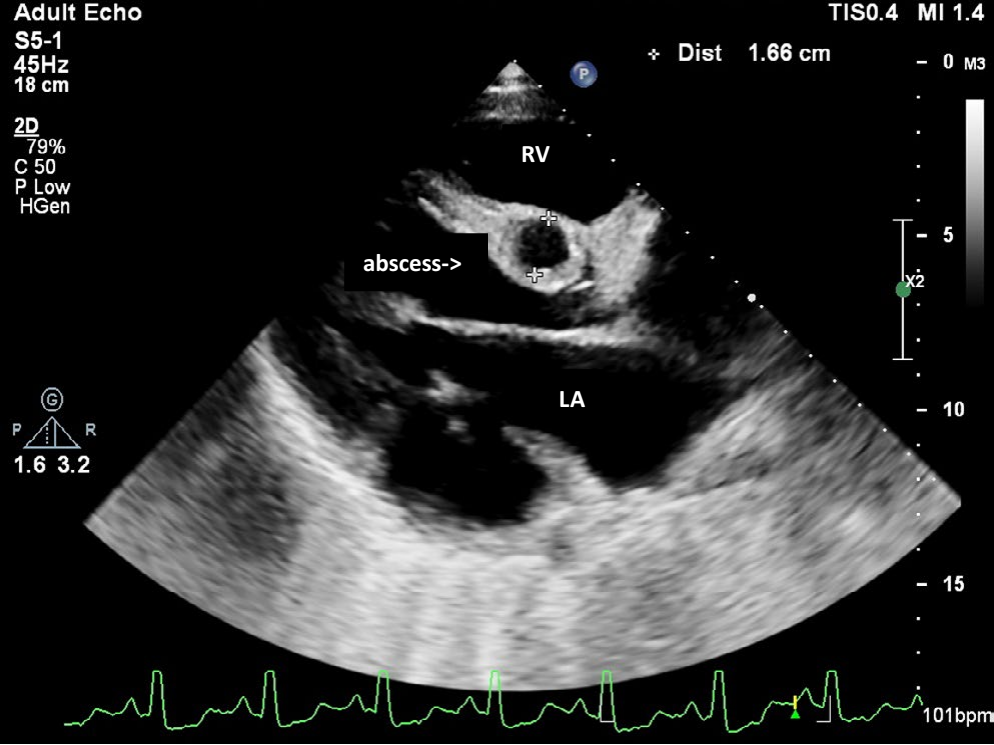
Long‐axis view of the transthoracic echocardiogram showing the interventricular septum abscess. LA, left atrium; LV, left ventricle; RV, right ventricle
